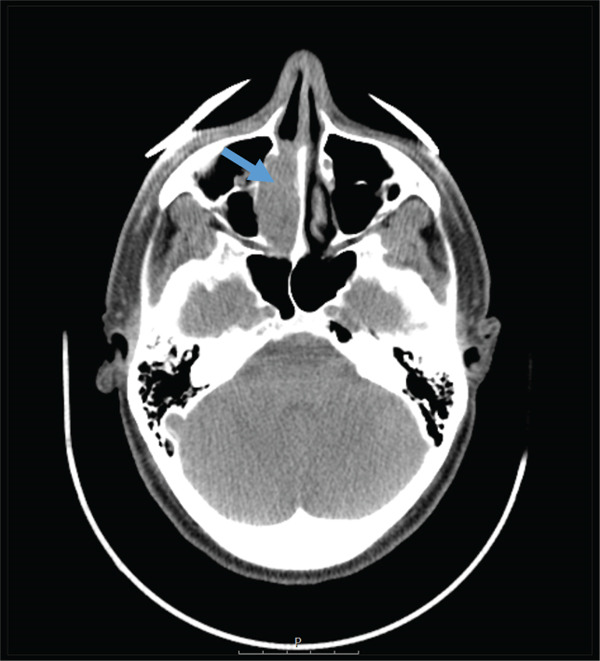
Axial CT scan of the brain findings consistent with a suspicious lesion of the papyraceous lamina with subocclusion of the nasal cavity (blue arrow).
